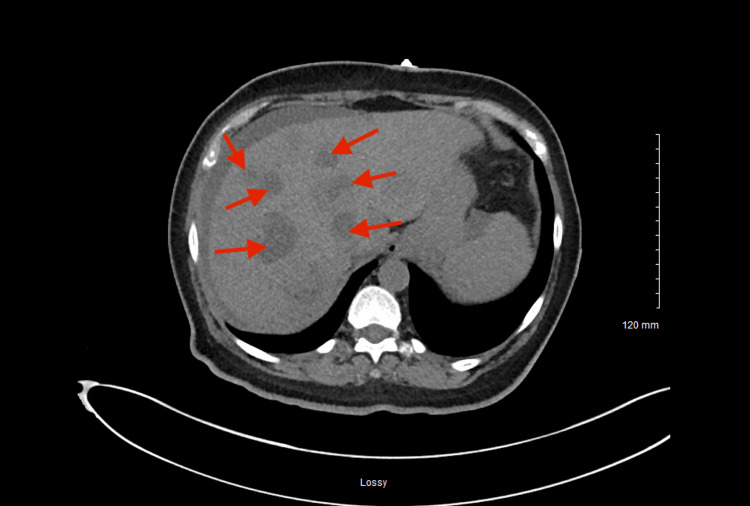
Contrast-enhanced CT of the abdomen and pelvis Contrast-enhanced CT demonstrates multiple hepatic lesions. Arrows indicate multiple hepatic metastases consistent with metastatic melanoma, establishing distant metastatic disease. CT, computed tomography
